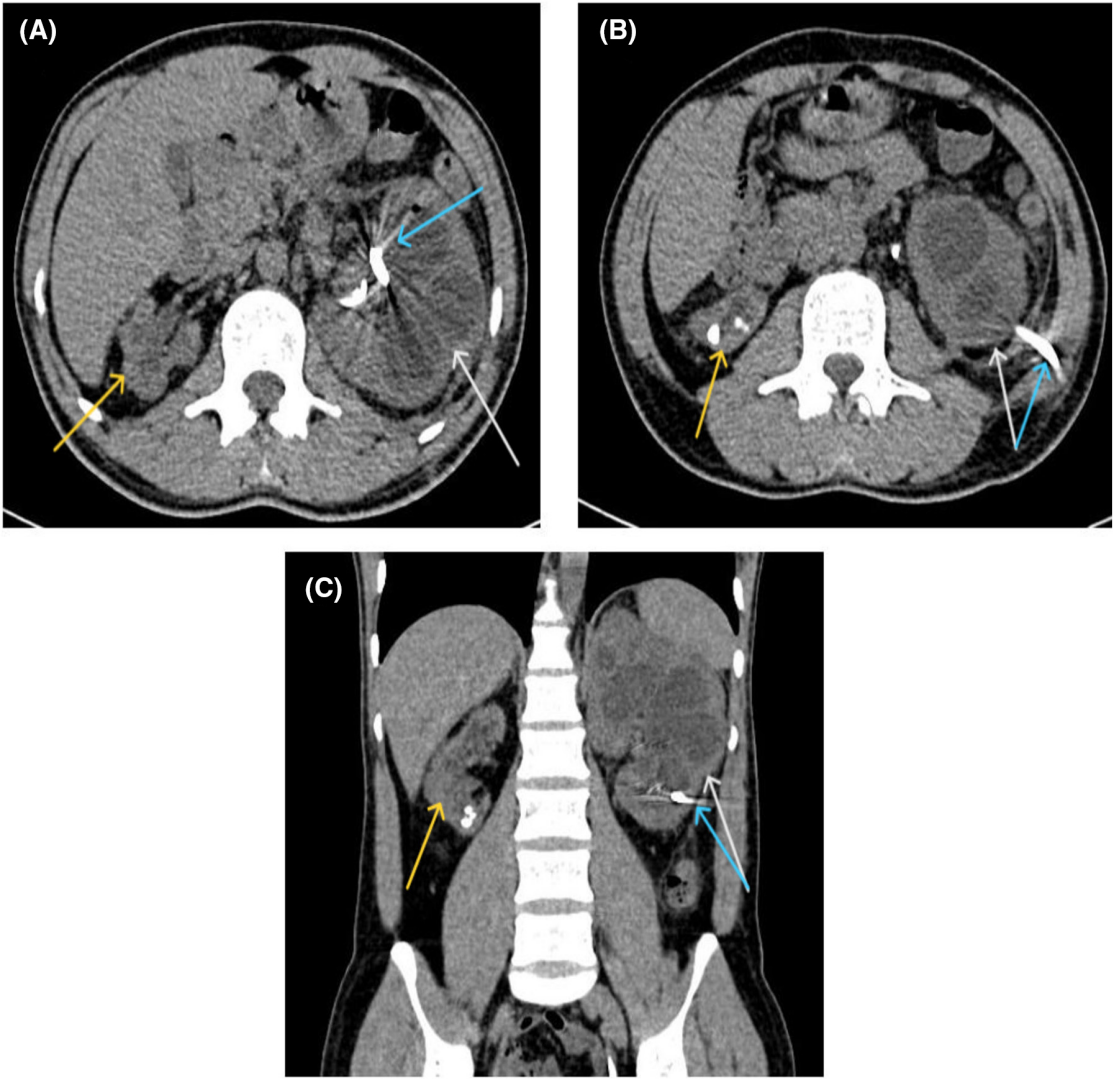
Axial (A, B) and coronal (C) views of the multi‐detector CT scan show enlargement of the left kidney mostly compatible with pyelonephritis (white arrow), the atrophic appearance of the right kidney with renal stones (yellow arrow), and a left‐sided nephrostomy and left double J catheter (blue arrow) at the time the patient presented with acute kidney injury.
